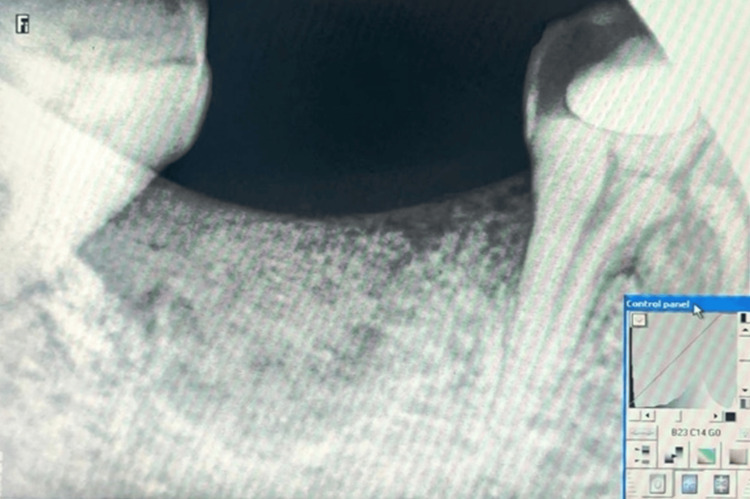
Radiograph after the placement of the graft
